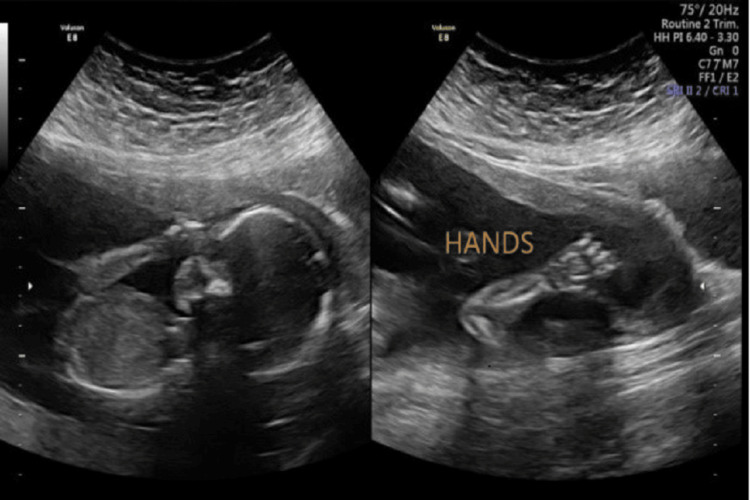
Ultrasound fetus on 11 weaker NT scan NT: nuchal translucency
